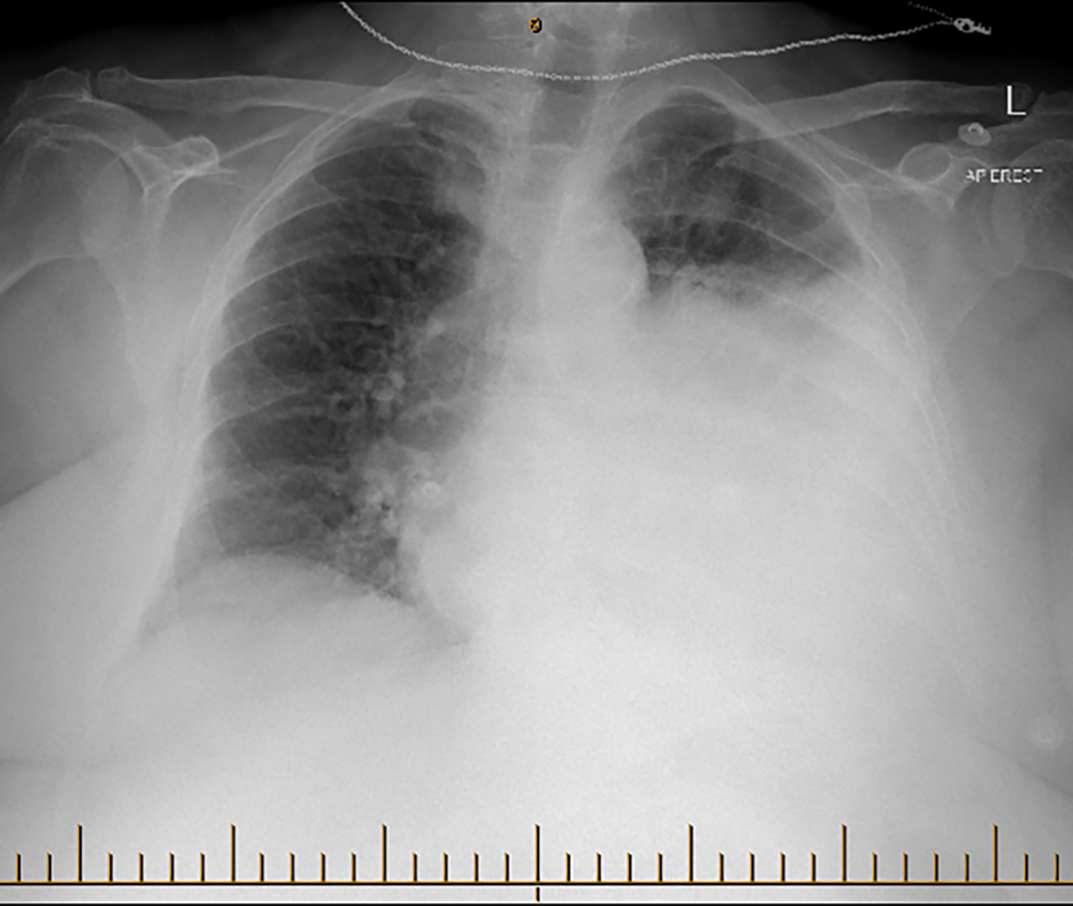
Chest radiograph on day of admission.
